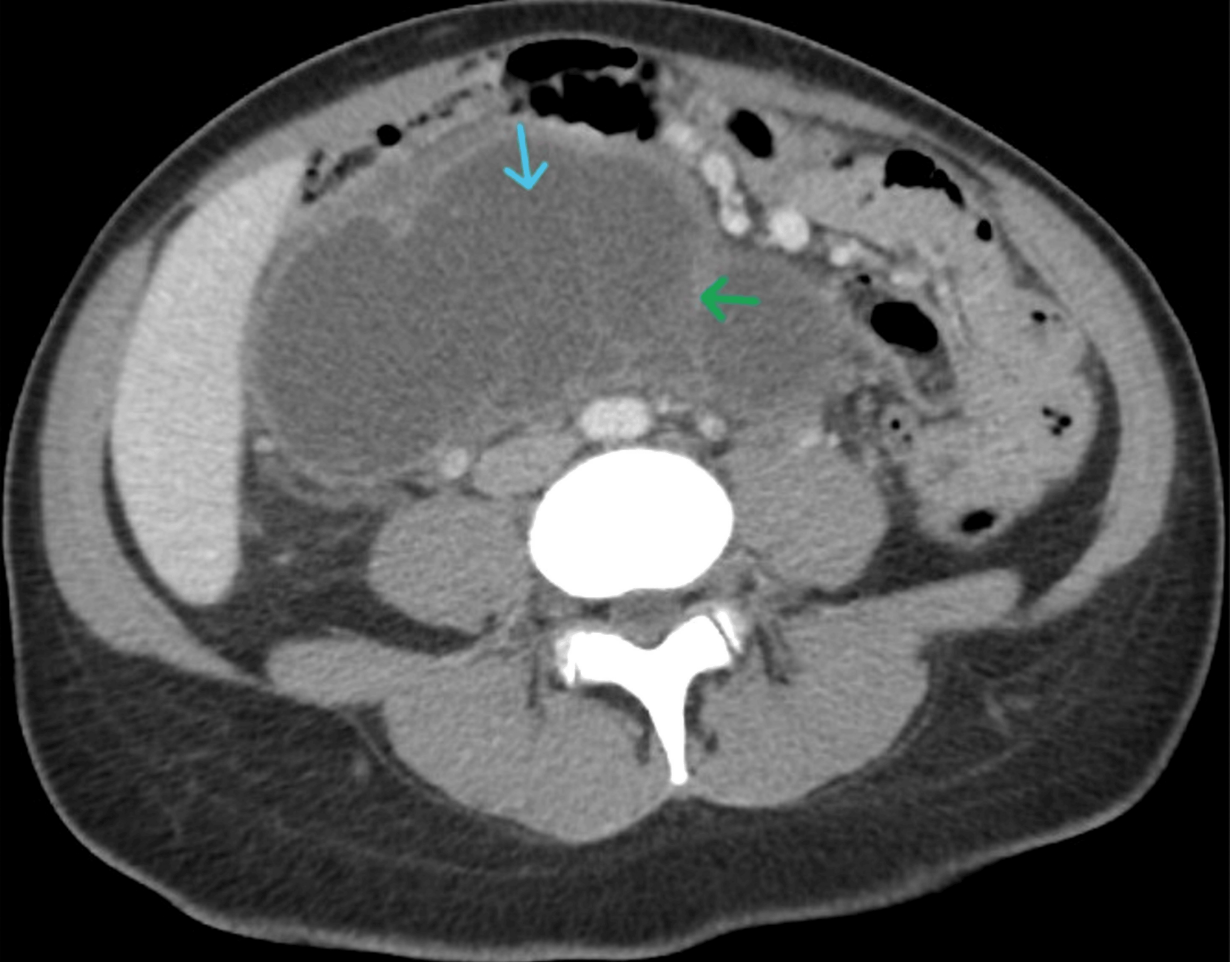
Contrast-enhanced CT of the abdomen and pelvis showing a large, complex, septate (green arrow), cystic (blue arrow) retroperitoneal mass The retroperitoneal mass is located in the mid-abdomen, extending from the right upper quadrant to just below the iliac crest, displacing the pancreas and duodenum anteriorly, and without a significant solid component. Mild enhancement is noted along the walls and septations.
